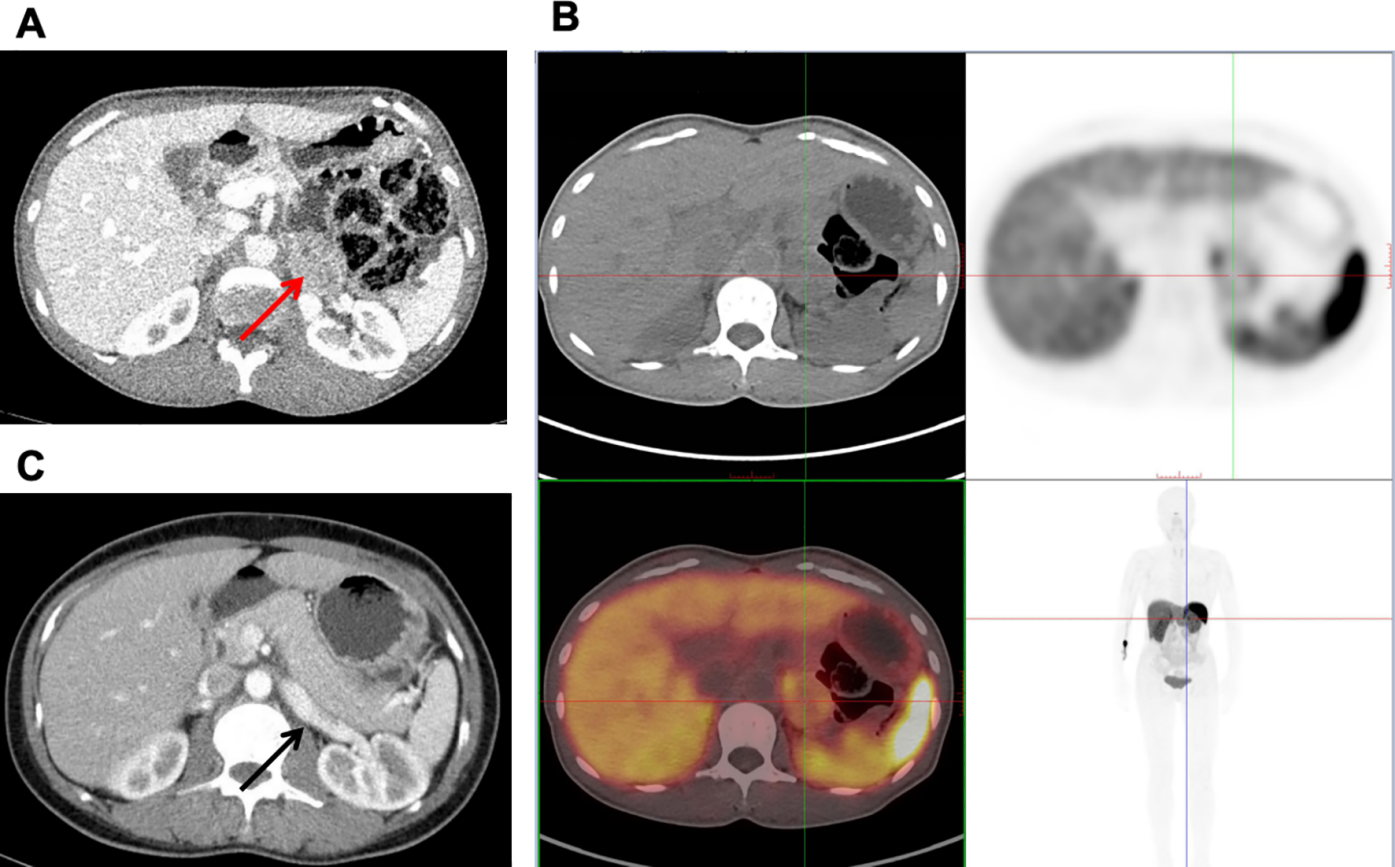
(A) Preoperative axial contrast-enhanced abdominal CT revealed an oval (44mm x 30mm), mildly enhancing soft-tissue lesion in the left adrenal gland (red arrow), suggestive of a pheochromocytoma. (B) Preoperative 68Ga-OCT PET/CT showed mild somatostatin receptor expression in the left adrenal mass, localized by crosshairs, indicative of a neuroendocrine tumor (NET). (C) Postoperative (3 months) axial contrast-enhanced abdominal CT demonstrated complete resection of the previous left adrenal tumor. The surgical bed shows no residual lesion or abnormal enhancement, consistent with postoperative change (black arrow).
